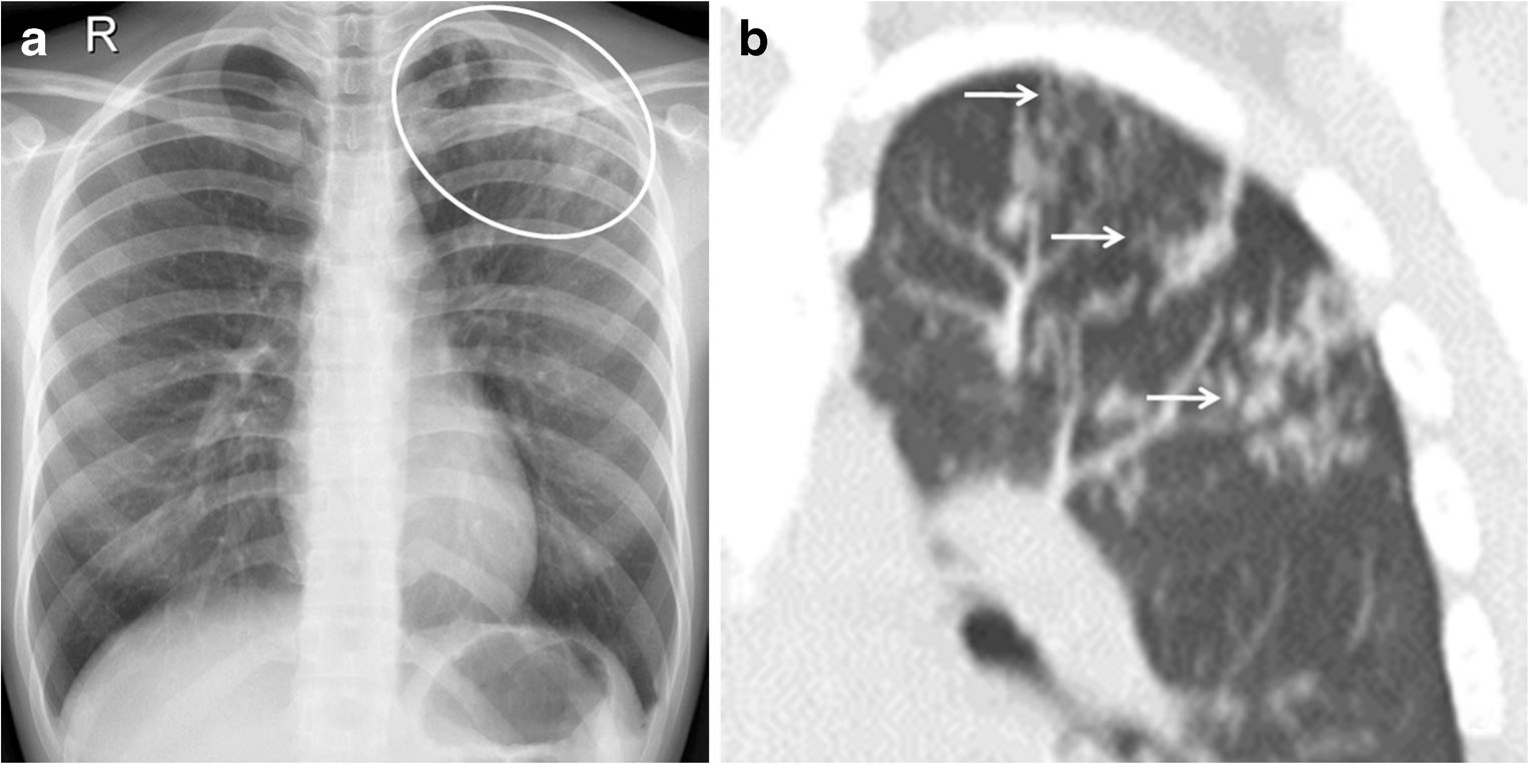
Post-primary tuberculous disease with bronchogenic spread in a 15-year-old girl. a Posteroanterior chest radiograph of shows ill-defined infiltrates in the left upper lobe (*encircled*). b Coronal reconstruction CT image demonstrates multiple centrilobular nodules (*arrows*) with linear branching opacities (tree-in-bud sign)
